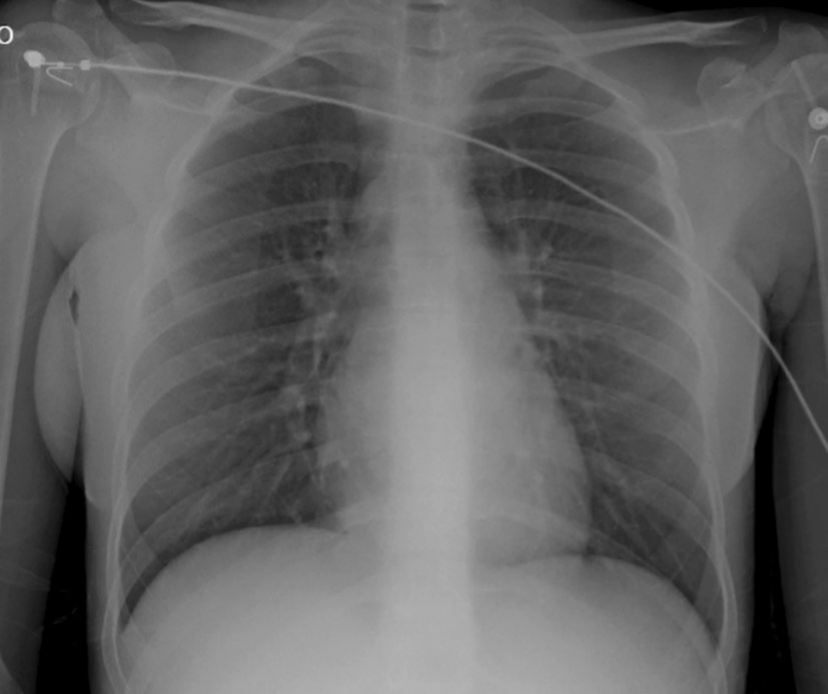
Chest X-ray at admission
